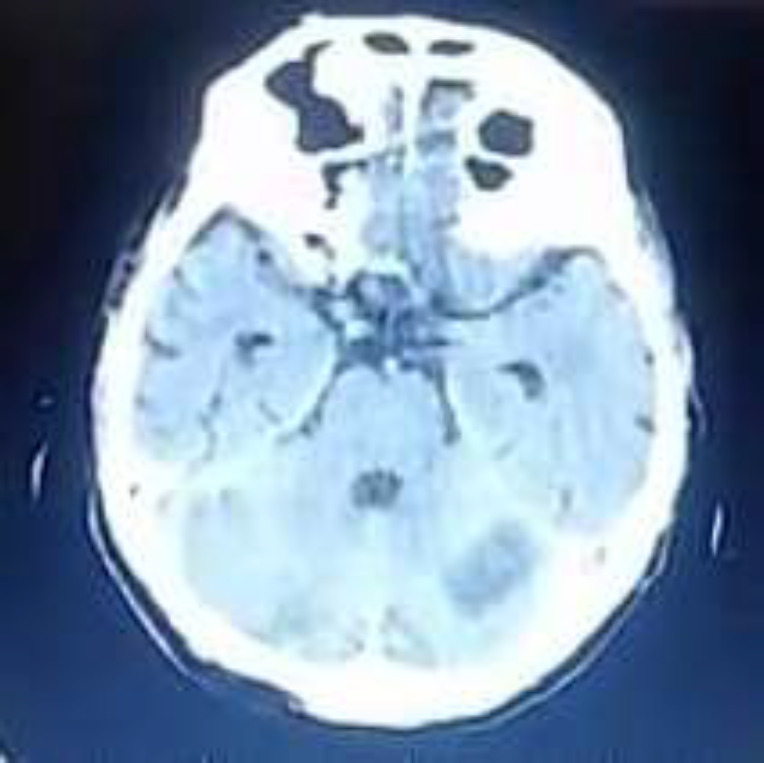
Axial unenhanced CT image of the brain showed hypodense lesion in the superior and lateral aspects of the left cerebellar hemisphere without significant mass effect
